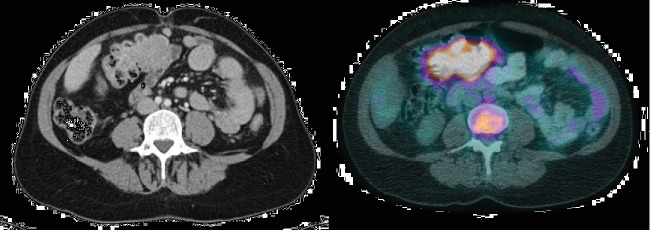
Transverse colon mass seen on abdominoplevic scan and PET-scan (FDG).
